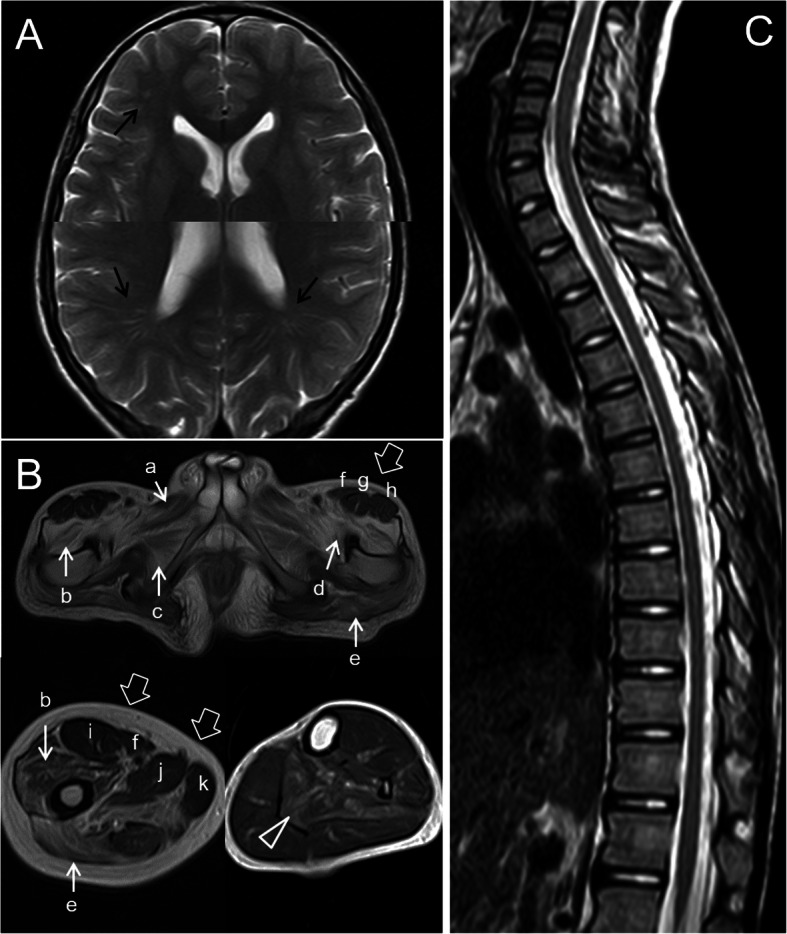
MRI of the brain, lower limb muscles and spinal cord. a Cerebral MRI showed abnormal signals in the subcortical white matter near the horns of the right lateral ventricle, which is high in T2-weighted imaging (black arrows), low in T1-weighted imaging and high in fluid attenuated inversion recovery (not shown). b The pelvic girdle and thigh muscles demonstrated obvious atrophy and fat infiltration of pectineus (a), vastus lateralis (b), iliopsoas (c), obturator externus (d) and gluteus maximus (e) (white arrows), while the sartorius (f), rectus femoris (g, i), tensor fascia lata (h), adductor longus (j) and gracilis (k) (white hollow arrows) were intact. Image of the legs revealed a moderate fat infiltration of the tibialis posterior (white hollow triangle). c The spinal cord did not exhibit atrophy
